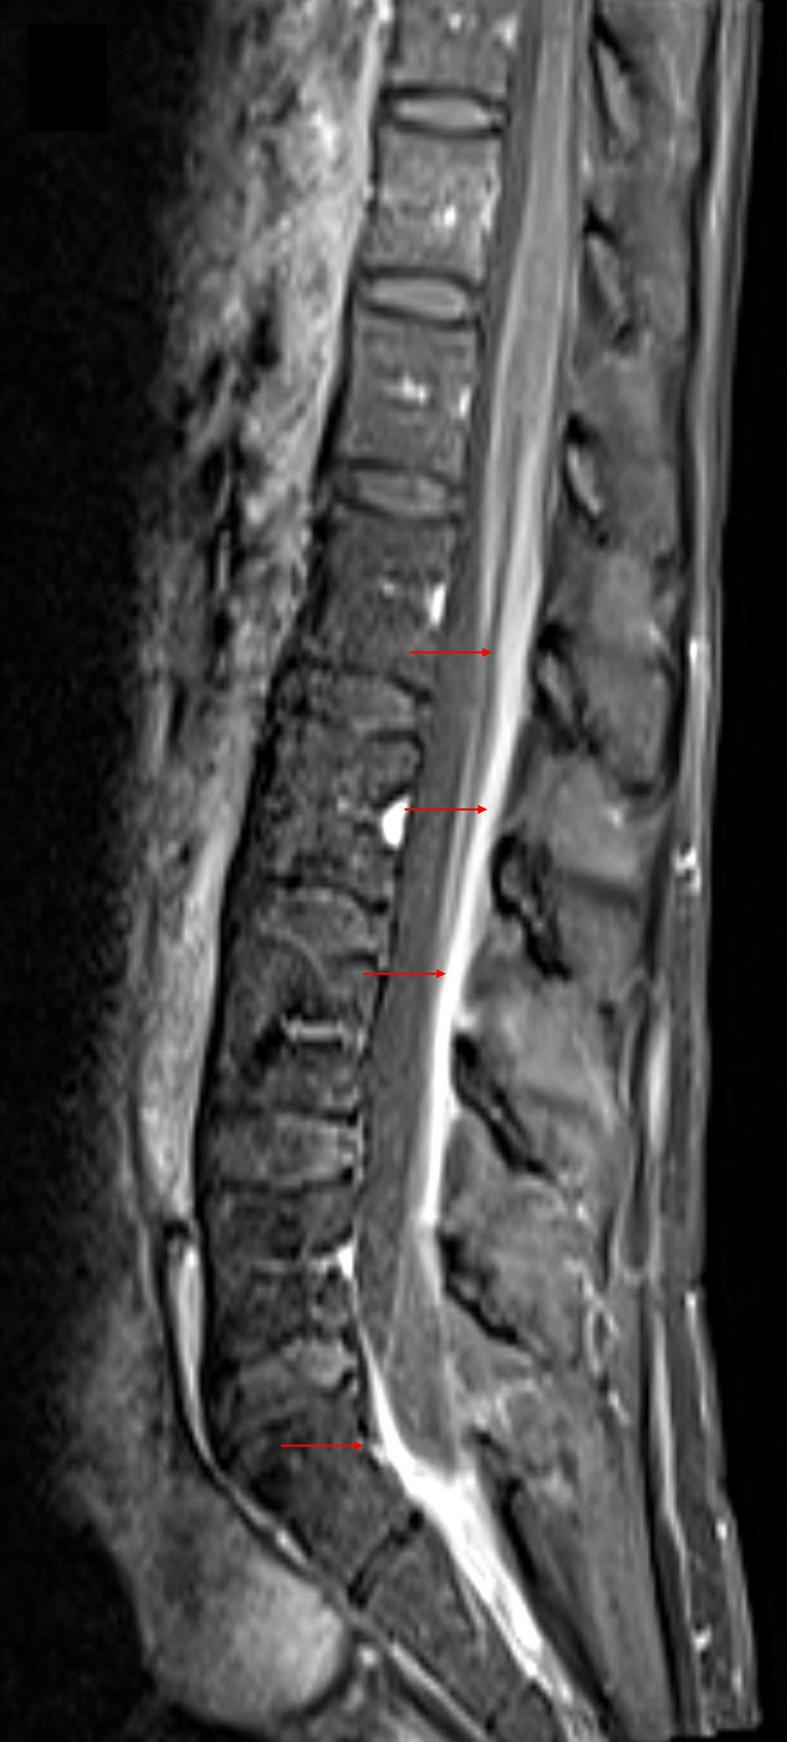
MRI of the lumbar spine revealing meningeal enhancement (red arrows). *MRI*, magnetic resonance imaging. (For interpretation of the references to colour in this figure legend, the reader is referred to the web version of this article.)
